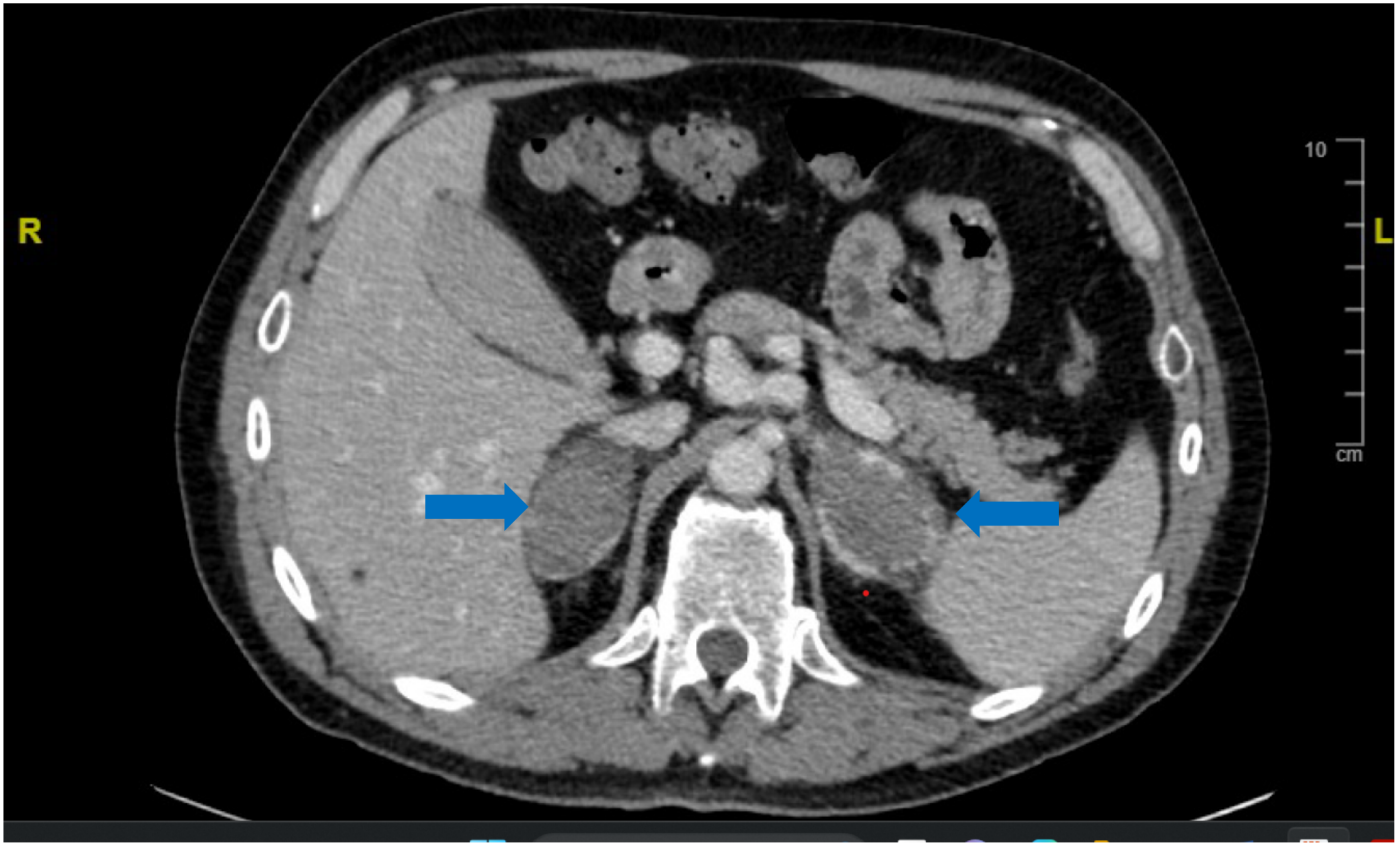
CT scan showing an increase of the size of both adrenals (blue arrows) with high density, suggestive for BAH, in case 2.
